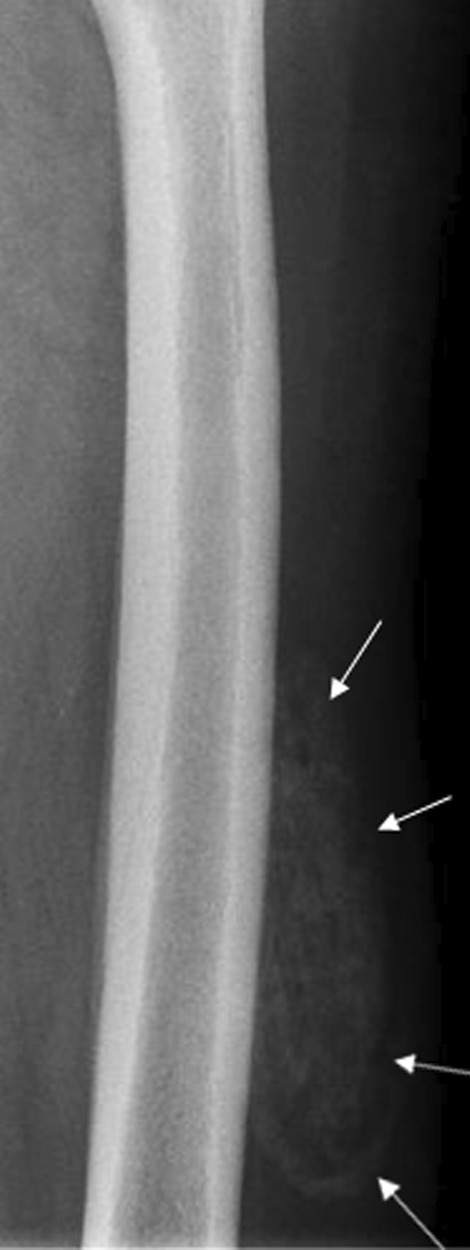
Plain radiograph showing a well-circumscribed mass with an ossified shell (arrows)
